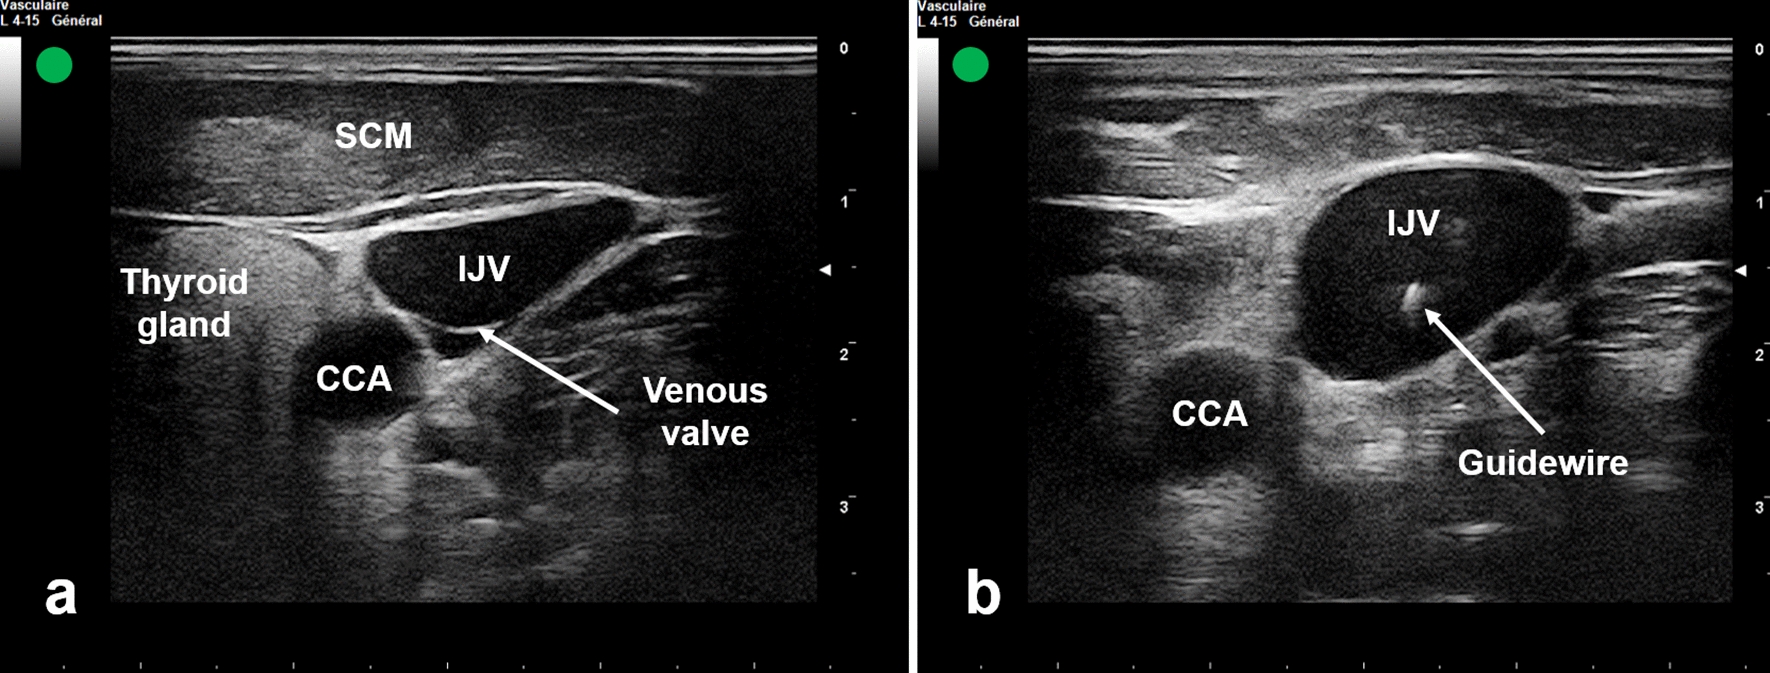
Ultrasound-guided IJV catheterization using the short-axis view of the vein in combination with out-of-plane needle approach. a Ultrasound visualization of IJV and CCA. b Ultrasound visualization of the guidewire in the IJV. *IJV* internal jugular vein, *CCA* common carotid artery, *SCM* sternocleidomastoid muscle
